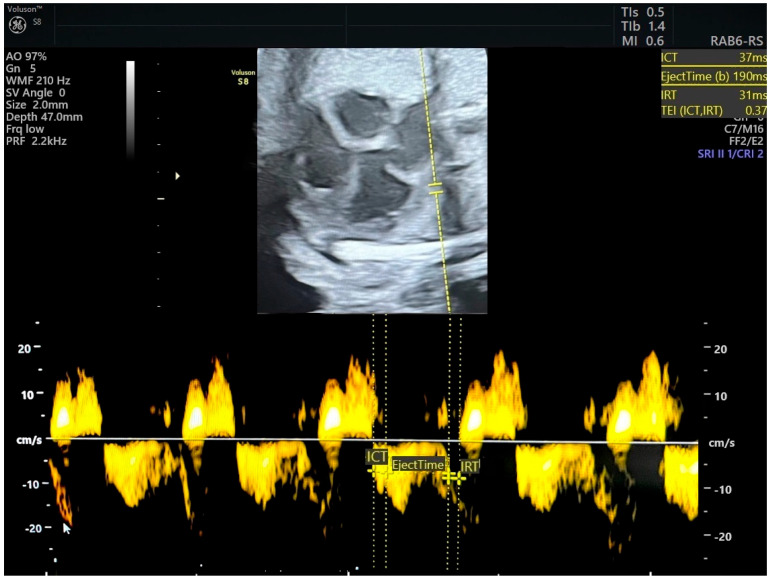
Pulsed tissue Doppler image of tricuspid annulus and measurement of right myocardial performance index. MPI was in normal range (ICT-isovolumetric contraction time, IRT-isovolumetric relaxation time, TEI = MPI). Source of image-personal collection.
